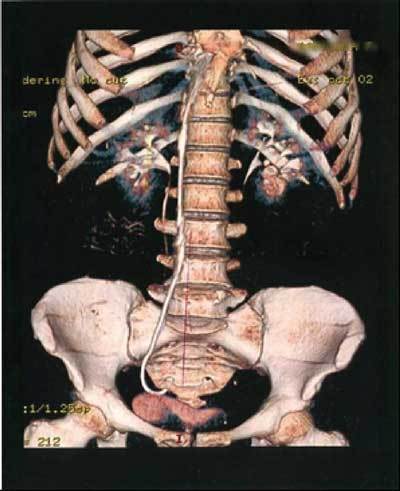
Computed tomography, 3D reconstruction, showing the catheter in the abdominal area.
